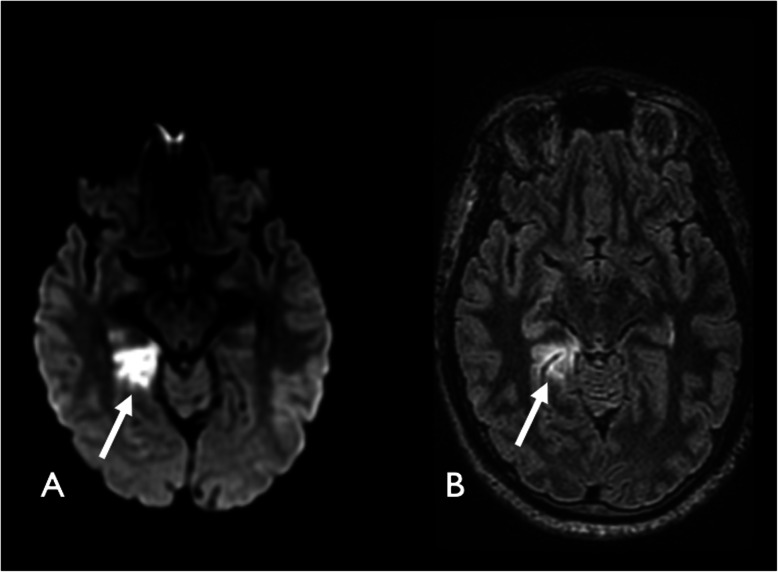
Axial diffusion (a) and FLAIR (b) MRI showing acute right posterior cerebral artery infarction (arrows)
